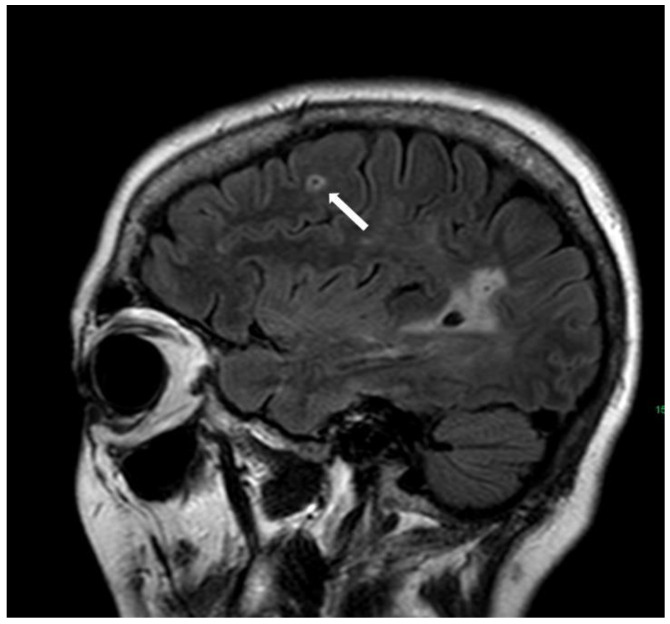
A lobar lacune in the frontal subcortical white matter with surrounding gliosis (arrow) on sagittal FLAIR (3 T, TE = 160 ms, TR = 12,000 ms, TI = 2850 ms, slice thickness 3 mm).
